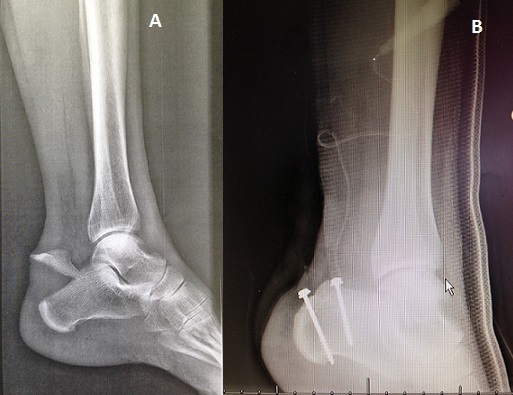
(A) radiologie standard de profile de la cheville gauche objectivant une fracture en bec de canard du calcanéum; (B) radiographie de contrôle de profile montrant l'ostéosynthèse par vissage de la fracture de clacaneum
